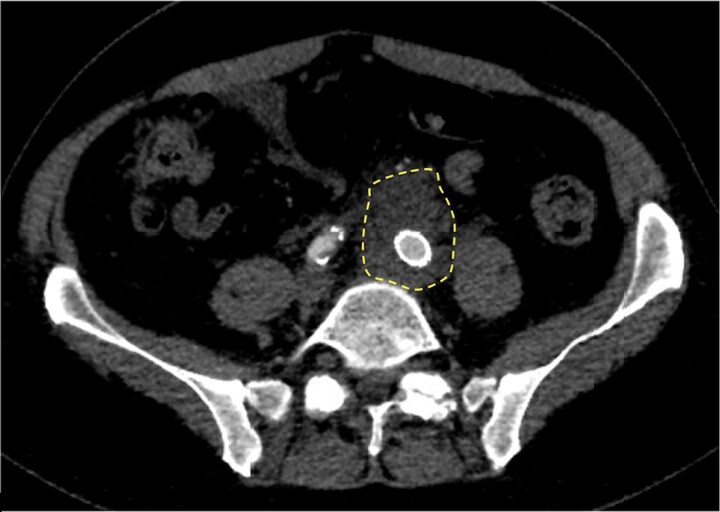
Case 1: axial cut of computed tomography angiogram shows soft tissue stranding around the left iliac limb of the endovascular aneurysm repair (dashed circle), suggestive of a thick phlegmon from a mycotic abdominal aortic aneurysm with an infected endograft.
